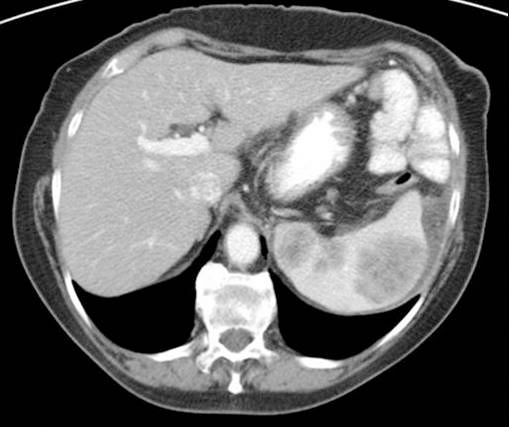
Computed tomography scan showing splenic masses.
